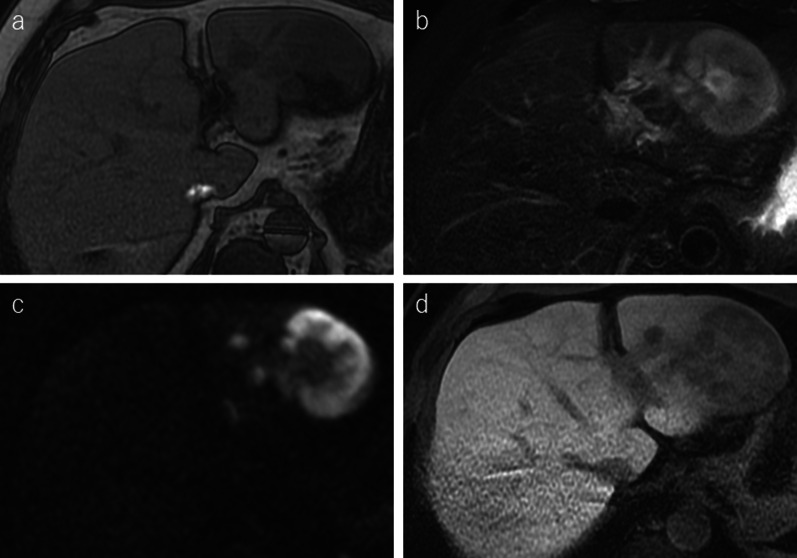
Magnetic resonance imaging (MRI) shows low intensity of the mass on T1-weighted images (a), high intensity on T2-weighted images (b), high intensity on diffusion-weighted images (c), and low intensity in the hepatobiliary phase of gadoxetic acid-enhanced MRI
